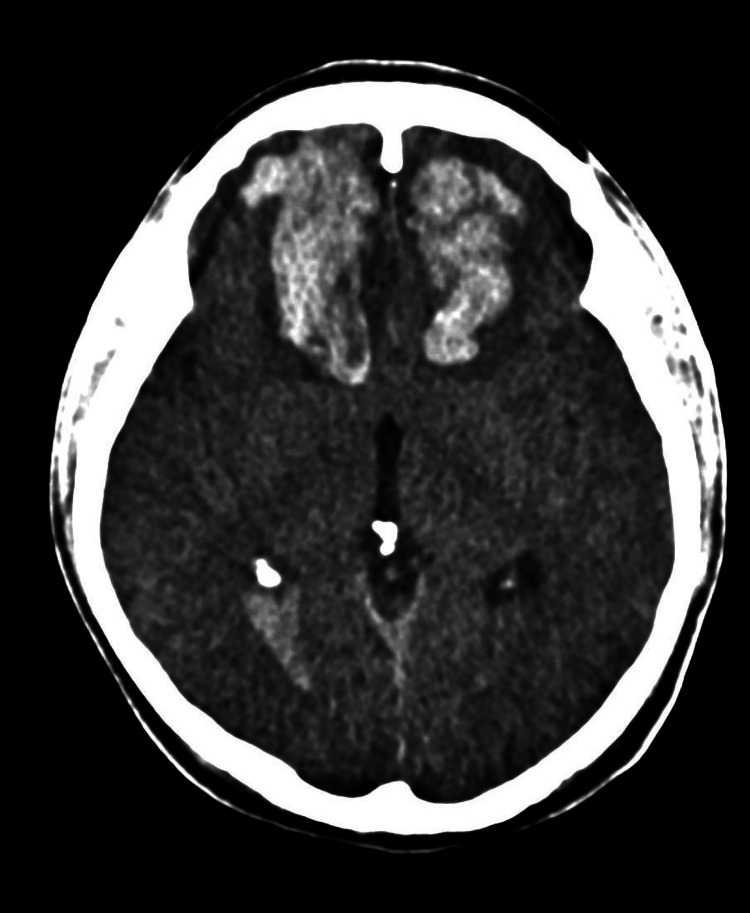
Head CT scan showing bilateral frontal intraparenchymal hematoma
